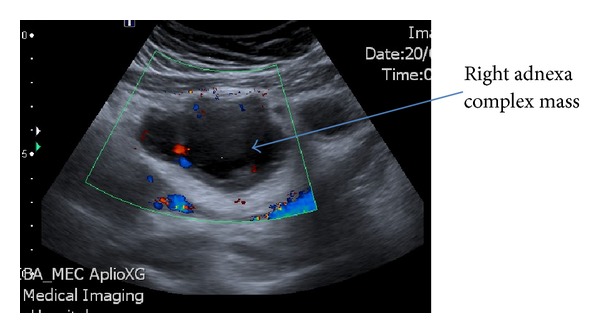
Transabdominal ultrasound of right adnexa-complex mass noted 70 mm × 45 mm × 42 mm.
